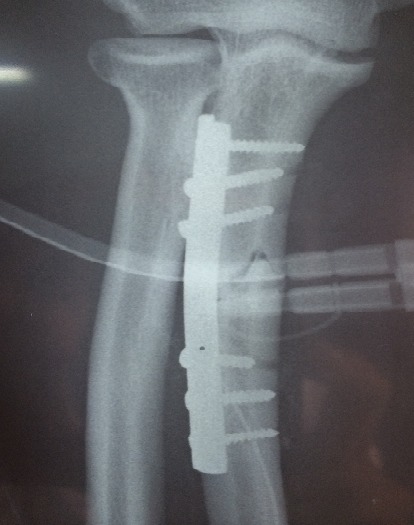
Radiography post operative control
